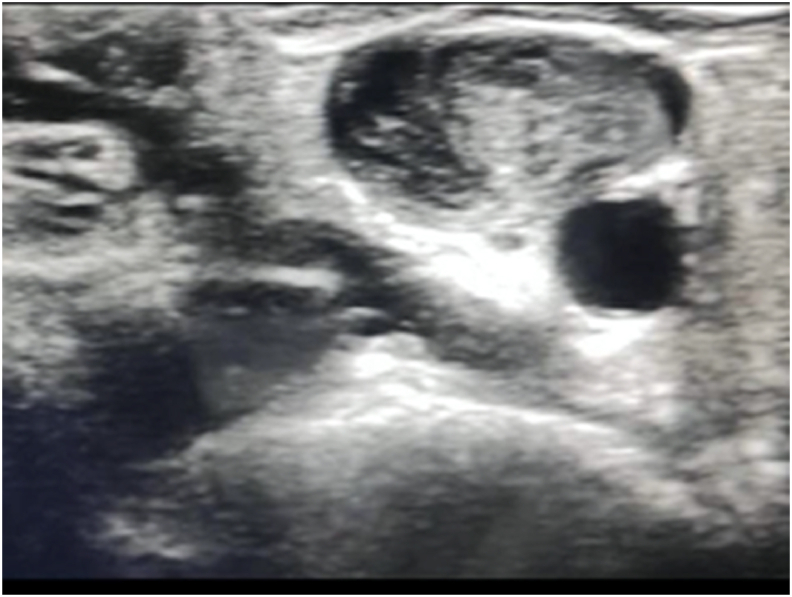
Ultrasound image of left internal jugular vein thrombosis.
